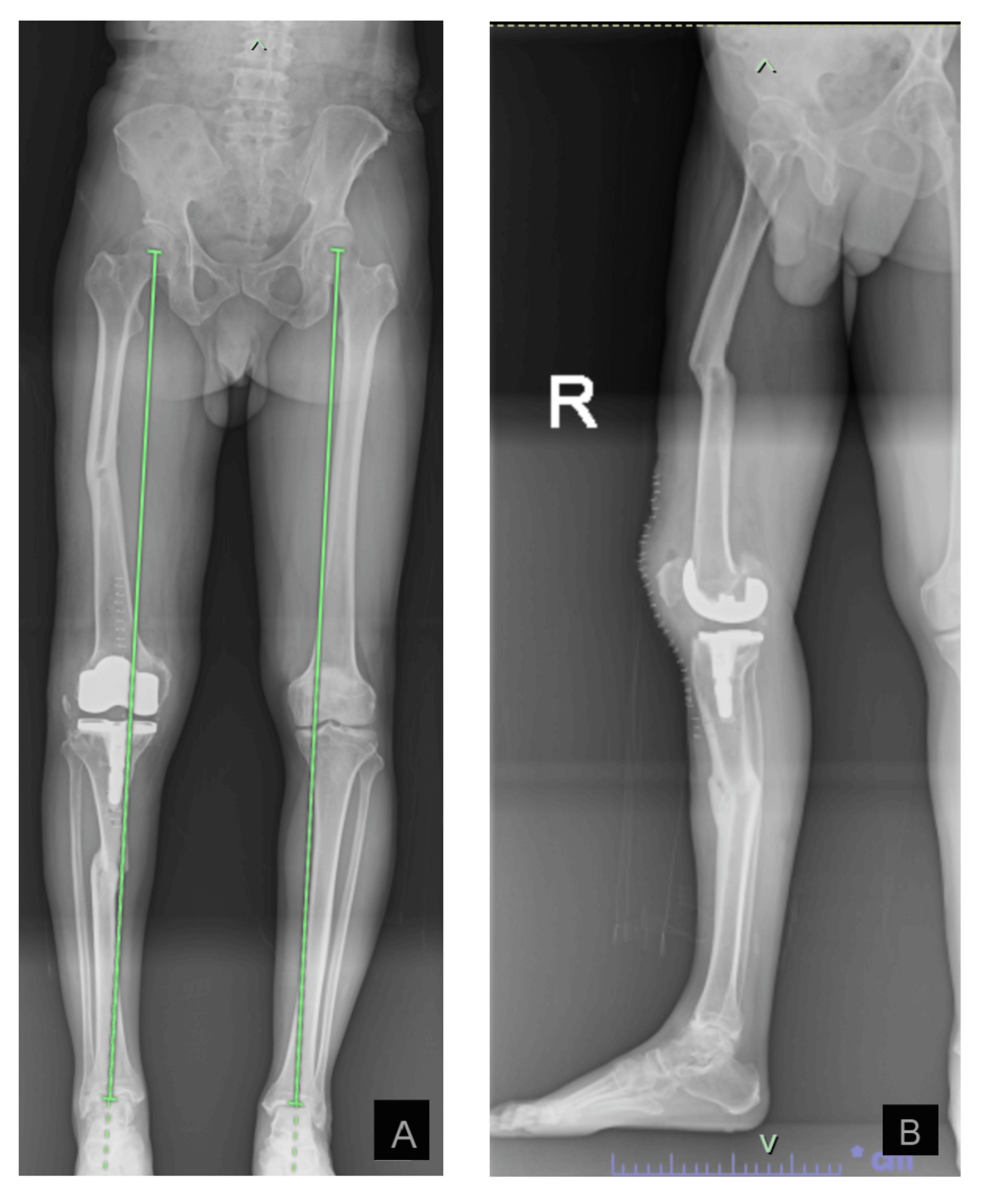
Post-operative radiographs. A) Anterior-posterior scanogram of the bilateral lower limbs and B) lateral view of the right lower limb Figure 2A is the anterior-posterior view of the bilateral lower limbs. The green line indicates the mechanical axis of the lower limb. The mechanical axis of the right lower limb is restored post-operatively. Figure 2B is the lateral view of the right lower limb. The sagittal alignment of the lower limb has improved post-operatively.
